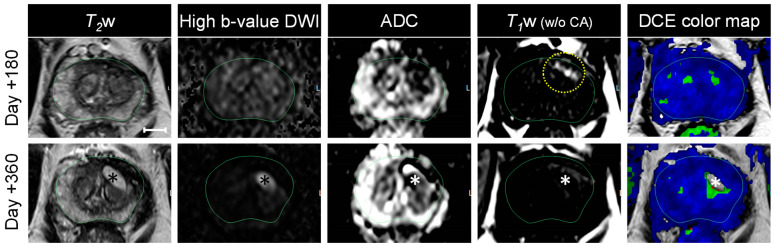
Six- and twelve-month follow-up mpMRI post-AuroLase^®^ Therapy. Axial mpMRI on Day +180 and Day +360, covering the *T*_2_w MRI, high b-value DWI, ADC map, *T*_1_w MRI (without the *T*_1_ contrast agent (CA) and background subtraction), and *T*_2_w MRI with the DCE color map overlay (from left to right, scale bar = 10 mm). The MRI slices at the two follow-up timepoints correspond with the slices presented at screening (Figure 1) and Day 5 (Figure 5) performed under a 3T external magnetic field scanner. Four core biopsies were taken from the treated lesion (left PZa) at six months and confirmed benign–negative for malignancy. Evidence of hemorrhage due to treatment was still evident on Day +180 mpMRI (dashed yellow circle) and entirely resolved at one year. At one year post-treatment, 12 standard-core biopsies were taken (6/6 of the biopsies taken from the left hemisphere of the prostate, including the treated lesion) were confirmed negative for malignancy with evidence of focal acute and chronic inflammation. Treatment-related cystic changes (*) appeared bright on *T*_2_w MRI and DWI (high b-value), dark on the ADC map and *T*_1_w MRI (*w*/*o* the CA), and a lack of enhancement on the DCE color map. From the right hemisphere, where no MRI-visible lesions were detected on screening, six biopsies were taken, in which one biopsy confirmed atypical small acinar proliferation (ASAP) at the right PZa, one biopsy confirmed PCa (ISUP GGG 1) at the right transition zone, and the remaining four biopsies were benign–negative for malignancy.
